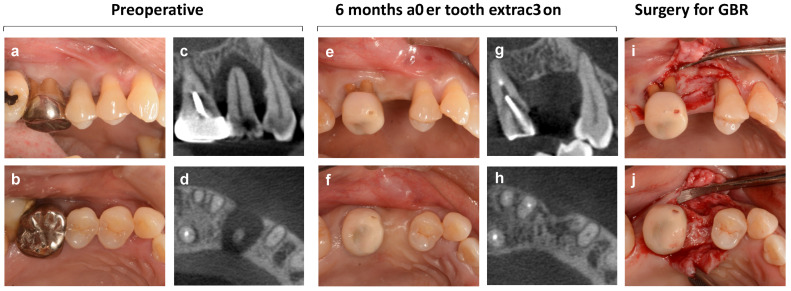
Natural healing and changes in the alveolar ridge after tooth extraction. (a,b) Intraoral photographs at the first visit. Maxillary right second premolar tooth (#15) is diagnosed as hopeless due to severe periodontitis, and then the tooth is extracted. Implant treatment was not initially planned. (c,d) Cone-beam CT images at the initial examination. (e,f) Intraoral photographs 6 months after tooth extraction. (g,h) Cone-beam CT images 6 months after tooth extraction. (i,j) Intraoral photographs during surgery of guided bone regeneration performed as pretreatment for implant treatment. The central portion of the alveolar ridge is cratered and depressed, and the buccal bone with malformed bony surface morphology is markedly reduced both horizontally and vertically.
